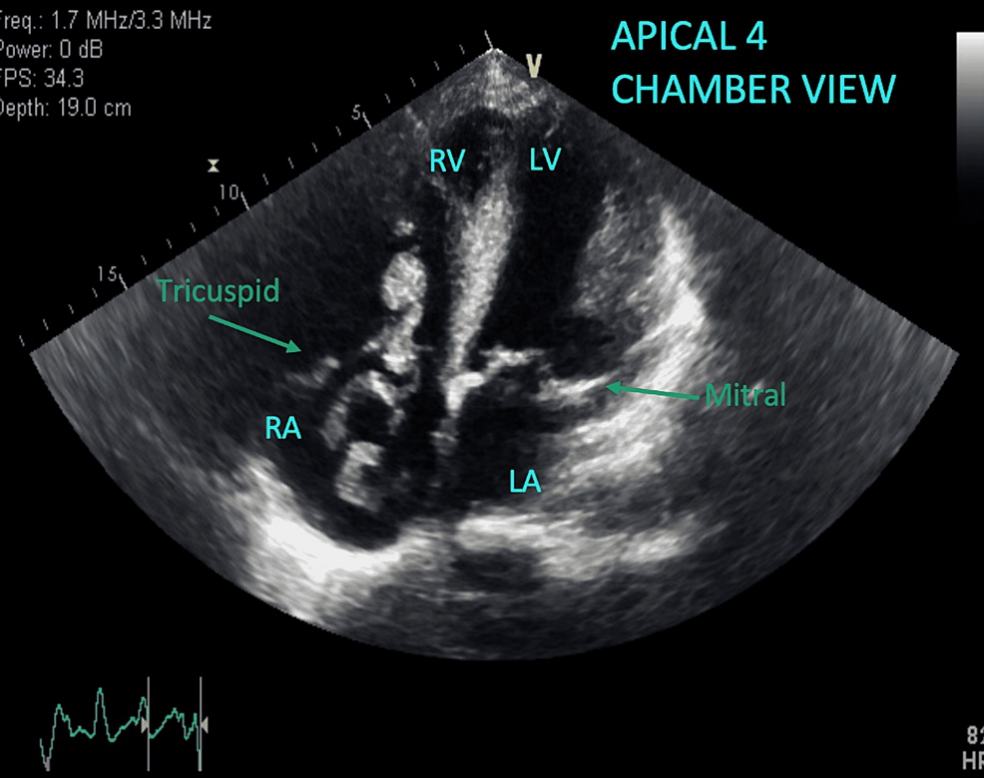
Apical four-chamber view visualizing the large, mobile, serpiginous mass (pulmonary embolus) regurgitating between the tricuspid valve. RV: right ventricle; LV: left ventricle; RA: right atrium; LA: left atrium
